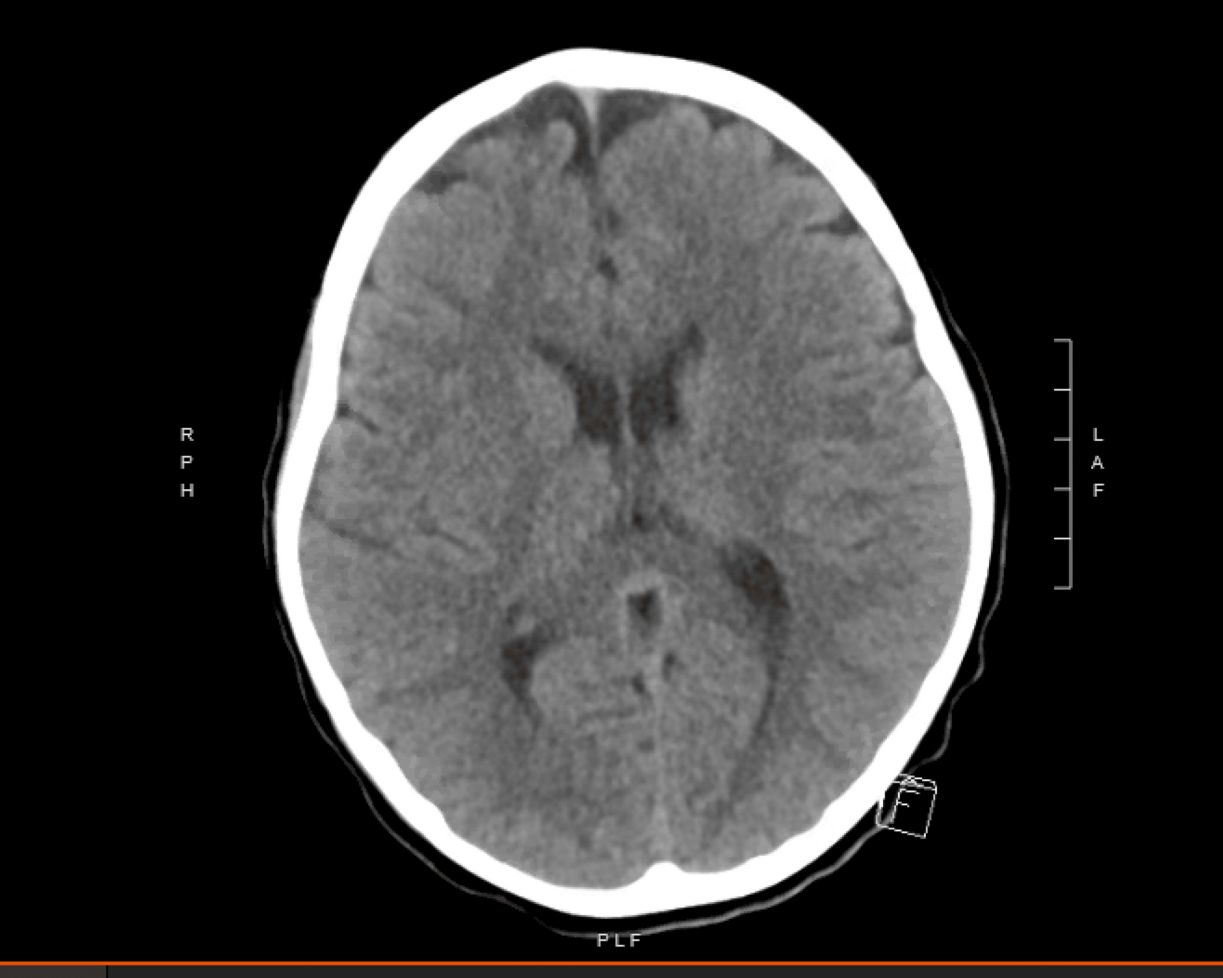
Patient's head CT CT: computed tomography
